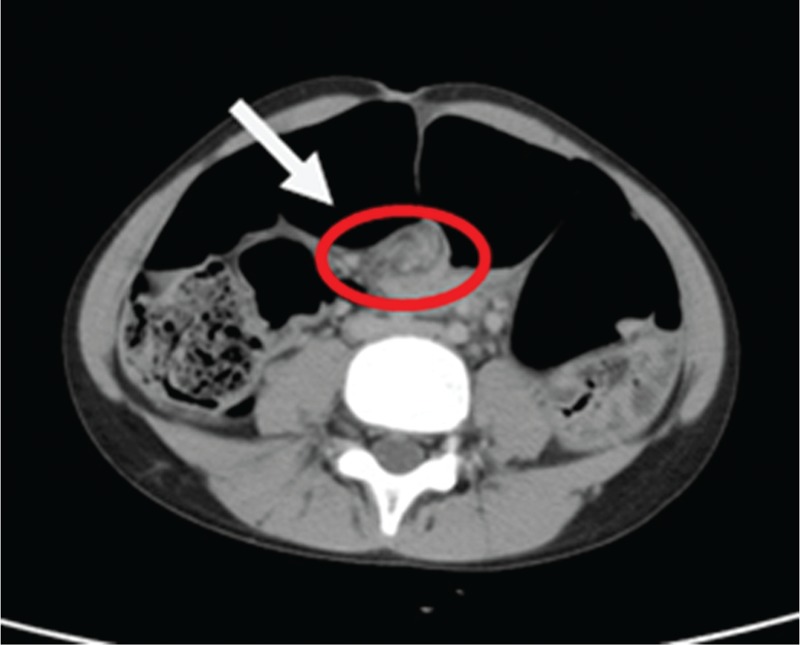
Whirlpool sign (arrow) with torsion of the sigmoid vessels in abdominal CT.
